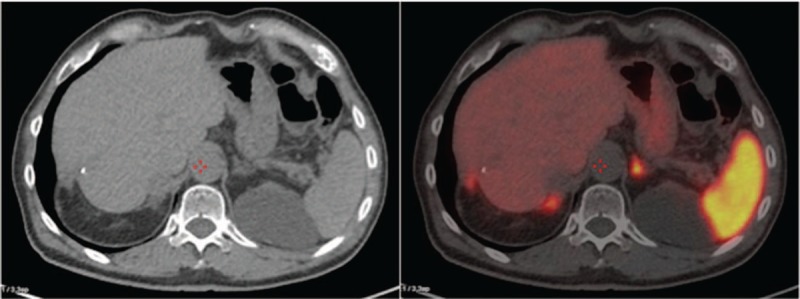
^68^Ga-DOTATOC PET/CT scan: as additional finding, in the medial lip of the left adrenal gland, surrounded by physiological uptake (SUV max 14.9), was found a nodular area suggesting adenoma (SUV max 27.5), confirmed by surgery.
